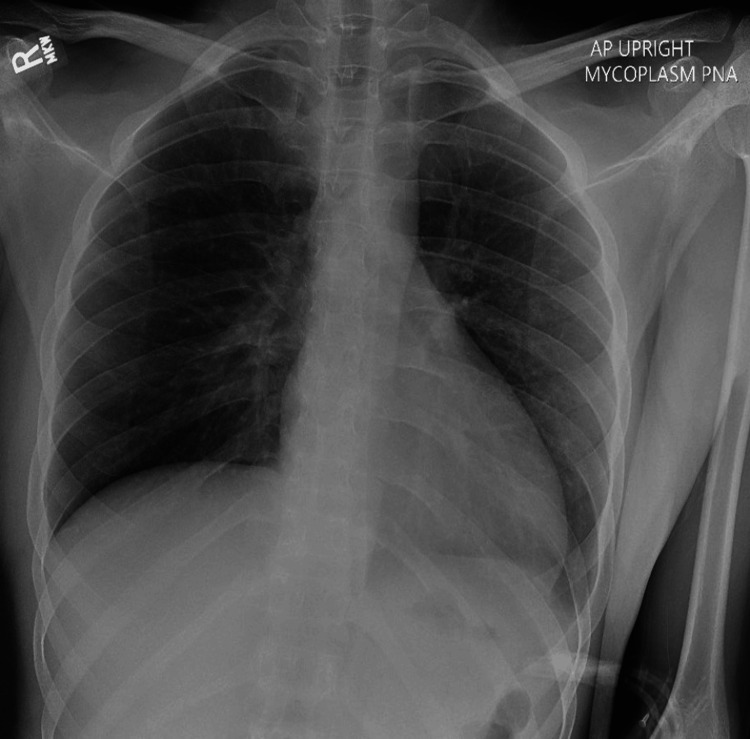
Chest X-ray five days after initial treatment
